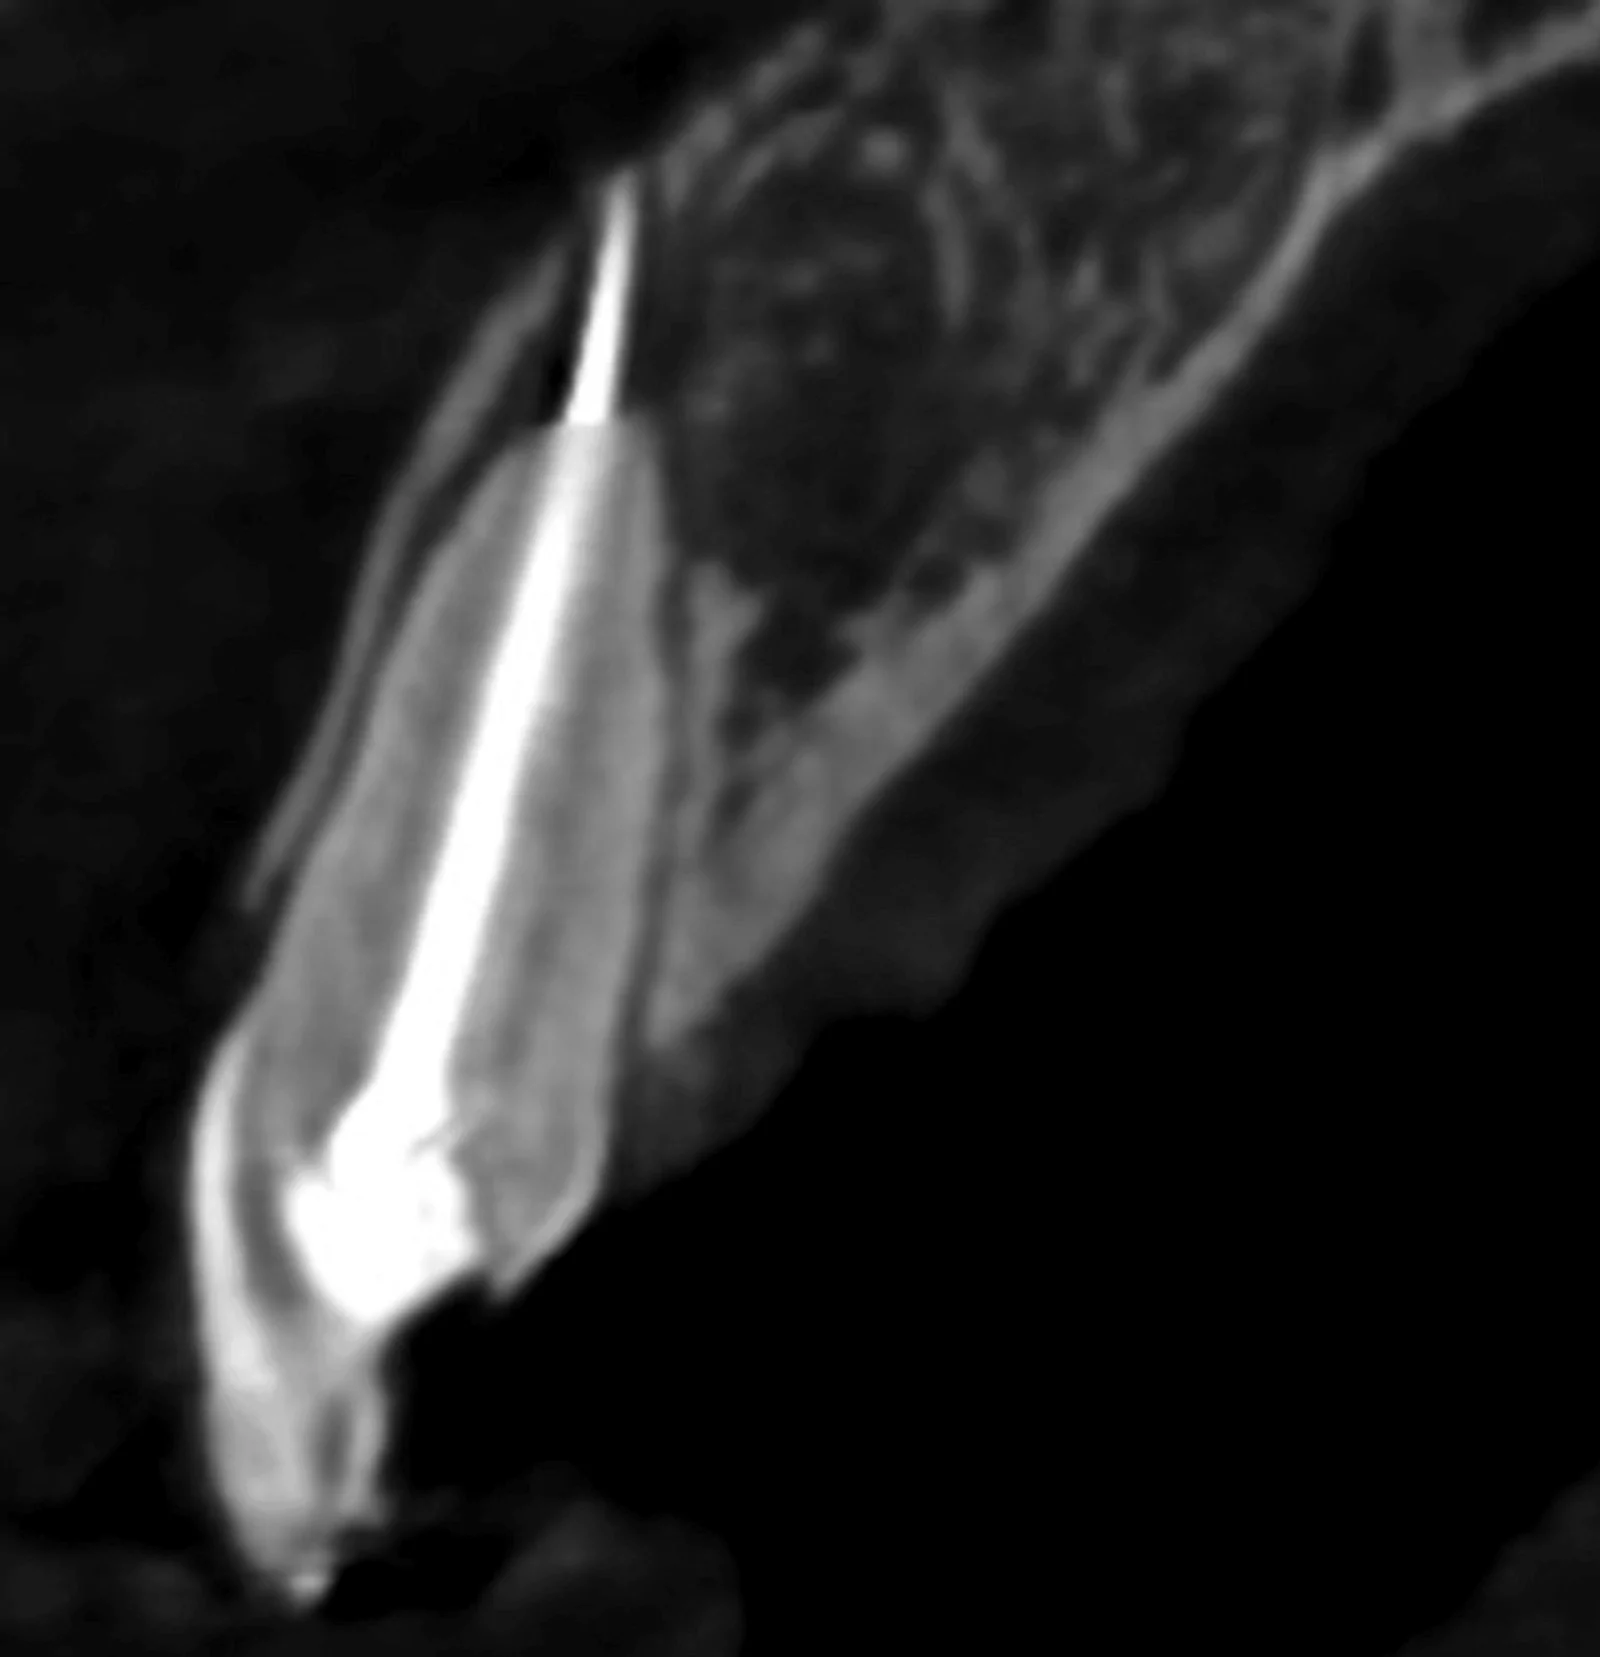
A periapical lesion at the apex of an endodontically treated tooth with an overfilling in the obturation material
